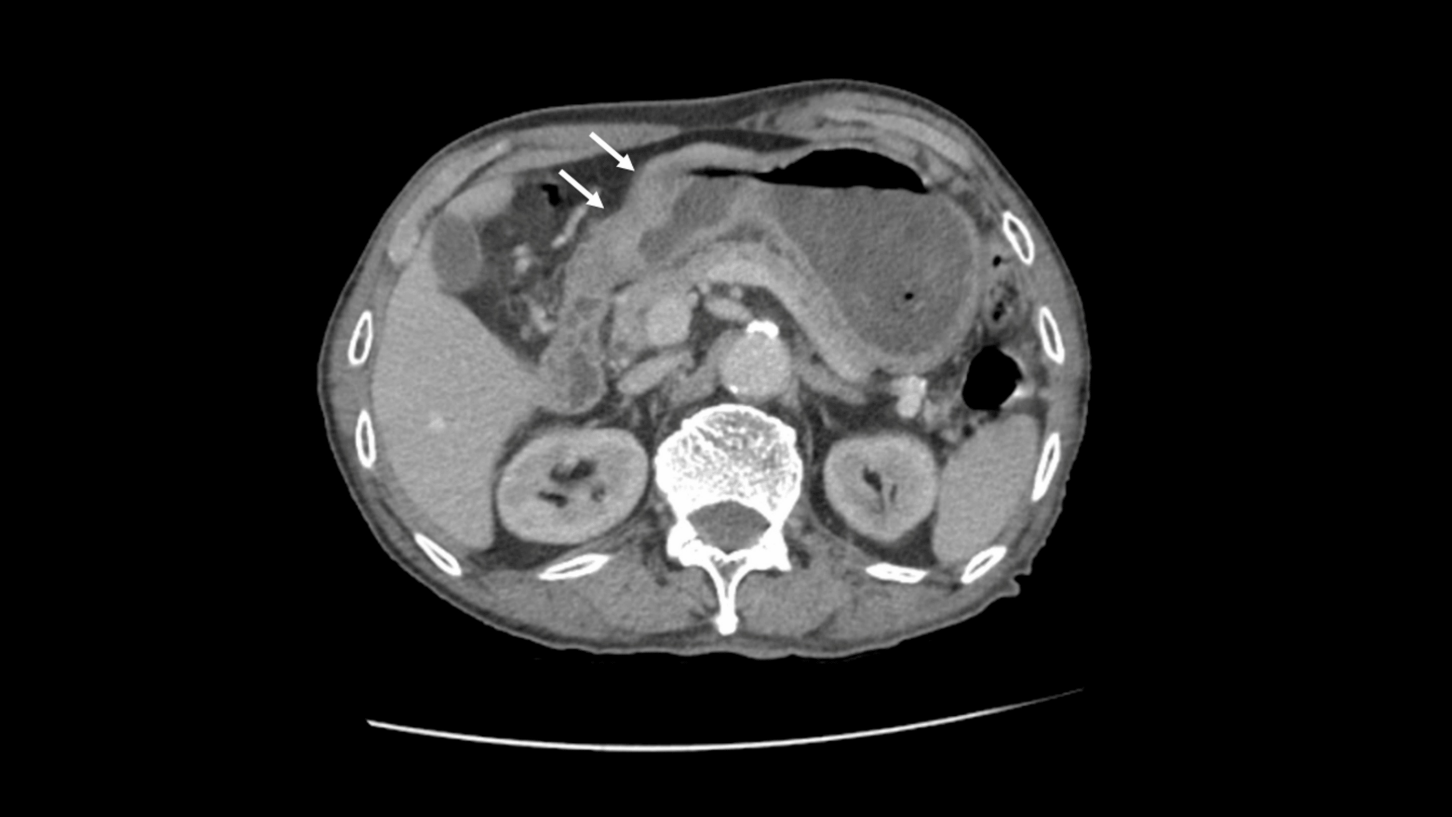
An contrast enhanced axial CT images showing thickened antrum affecting the anterior wall (arrows). CT: computed tomography
